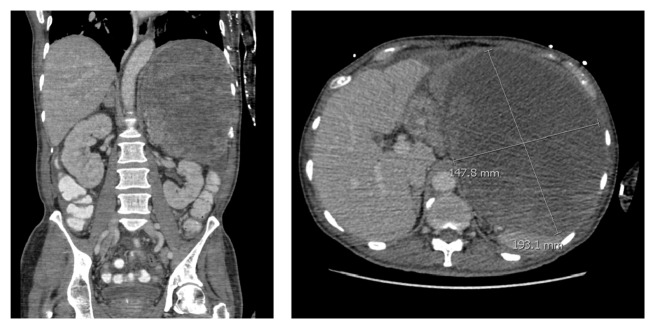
Abdominal CT scans demonstrating a 20 cm mass in the left upper quadrant retroperitoneum.
